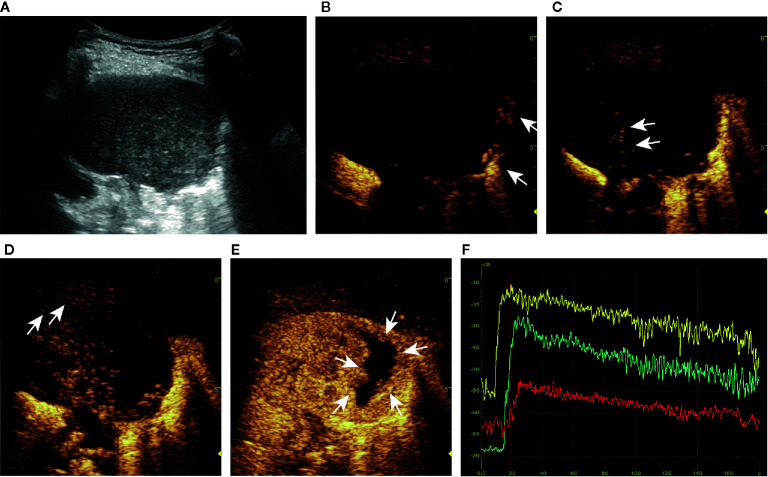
A series of ultrasound images of a malignant subpleural pulmonary lesion (squamous cell carcinoma) in the upper lobe of left lung of a 75-year-old man. B-mode ultrasound (A) showed the largest section of the lesion, and the angle between lesion border and thoracic wall was obtuse. The ultrasound contrast agent arrived at air-filled lung tissues (arrow) at about 9 s (B), the lesion (arrow) at 14 s (C) and the thoracic wall (arrow) at 18 s (D). At about 24 s, the lesion was completely enhanced and the intensity reached the peak (E). There was no obvious vascular sign in the lesion (C, D), and the non-enhancing region (arrow) was irregular (E). Accurate quantitative CEUS parameters can be obtained from the time-intensity curves of air-filled lung tissues (yellow curve), lesion (green curve), and the thoracic wall (red curve) (F): basic intensity = -68.22 dB, lung-lesion arrival time difference = 6.02 s, ratio of arrival time difference = 65.86%. The malignant probability calculated by the ultrasound prediction model was 98.42% > 45.59%, so the lesion was predicted to be malignant, which was consistent with the definite diagnosis. The corresponding dynamic clip is shown in Supplementary Video 2.
